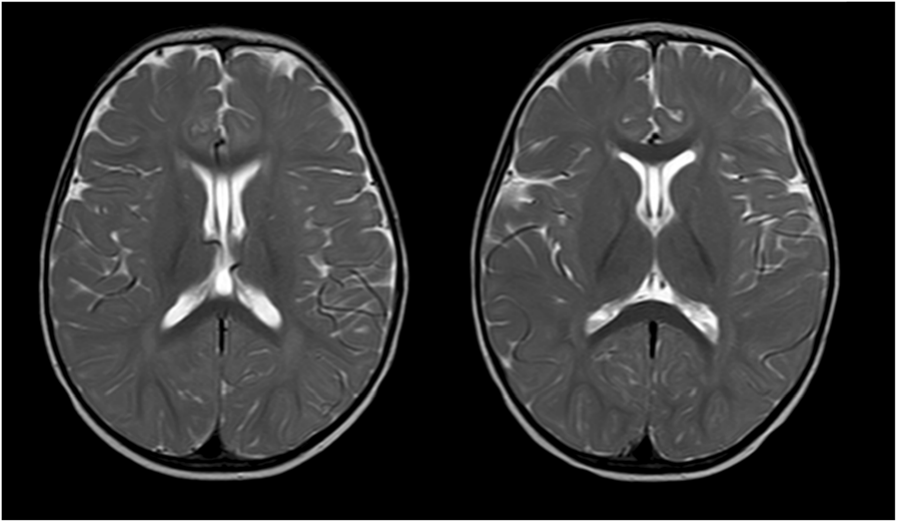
Brain magnetic resonance images at 14 months of age. The images show delayed myelination for this age
